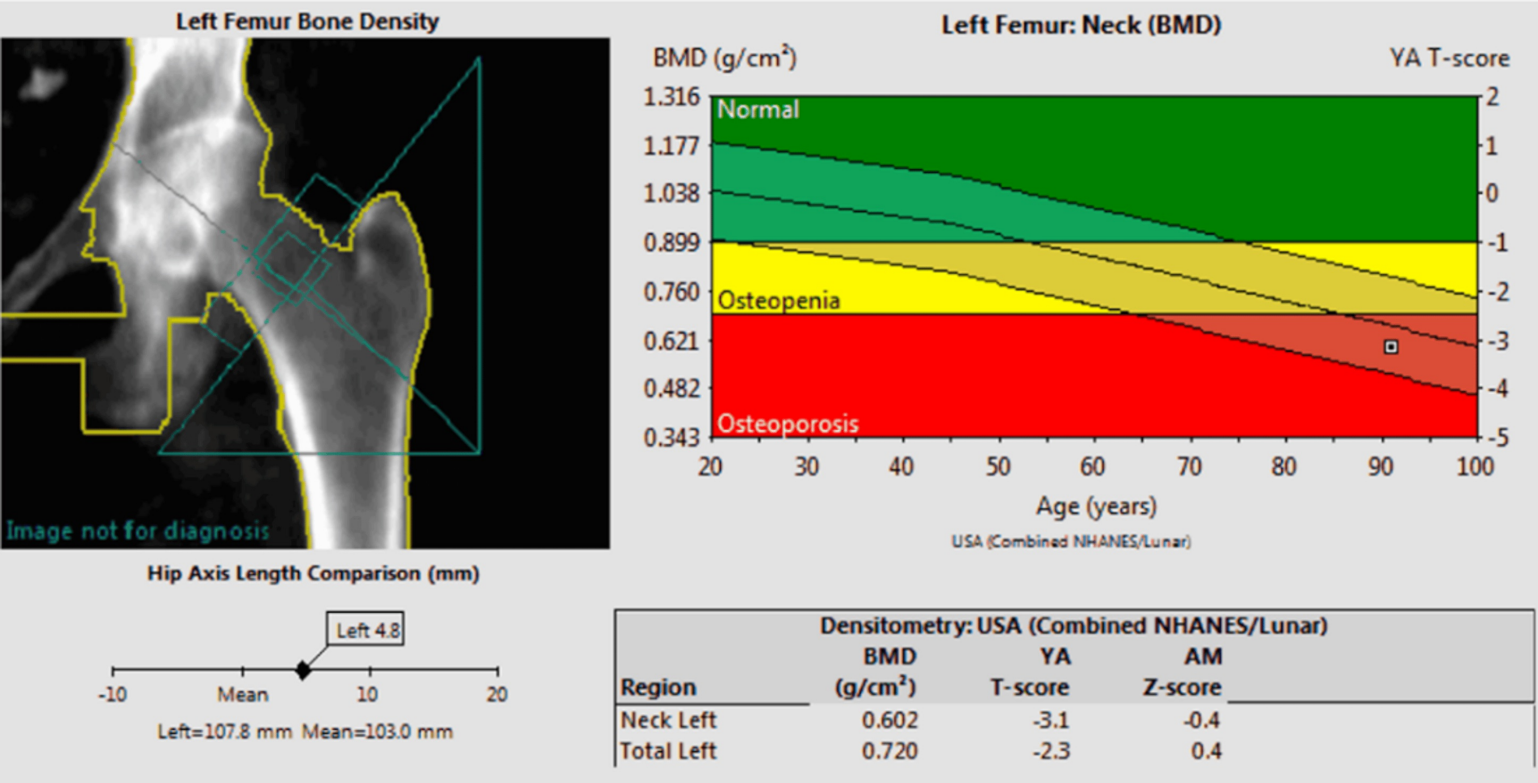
Pretreatment DEXA DEXA: Dual-energy X-ray absorptiometry; BMD: bone mineral density DEXA scan images obtained in 2020 of the left femoral neck reveals a BMD 0.602 and a T-score of -3.1
